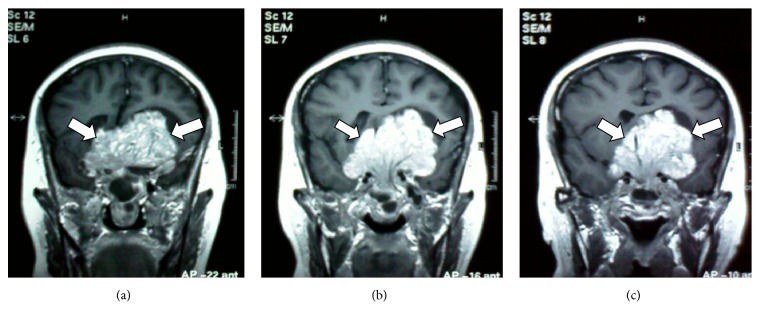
Initial contrast enhanced coronal T1-weighted sequence on MRI showing a large lobulated enhancing solid mass in the sellar and suprasellar regions (white arrows). The mass extends into the subfrontal lobes and in between the two frontal horns causing compression of foramen of Monroe. Inferiorly the mass extends to the sphenoid sinus and into the nasal cavity. The mass appears to encase the cavernous sinus and the optic chiasma.
